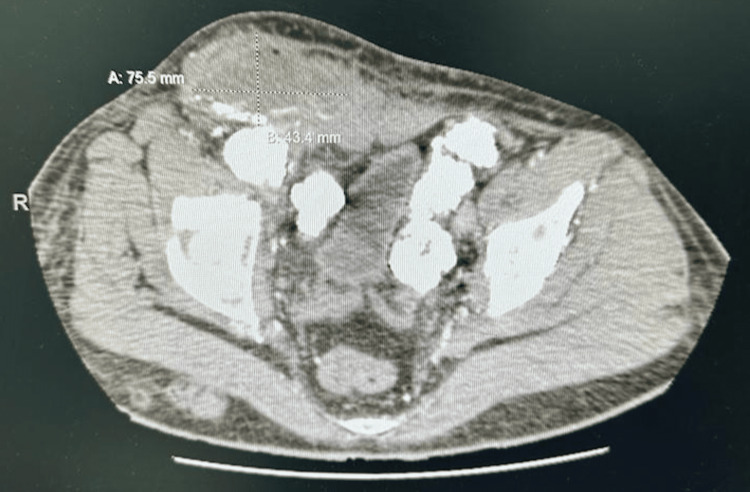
CT angiography of lower extremities The findings redemonstrated the diffuse extensive amorphous vascular/perivascular calcifications. Heterogenous irregular soft tissue collection seen in close contact with the right femoral artery and veins presently measured approximately 7.5 cm x 4.3 cm with minimal contrast extravasation. Few air foci were noted within, which could be due to superimposed infection. However, bilateral common iliac arteries and both posterior tibial arteries appeared normal. There was an inability to assess the latency of the rest of the lower limb arteries due to extensive wall calcifications.
